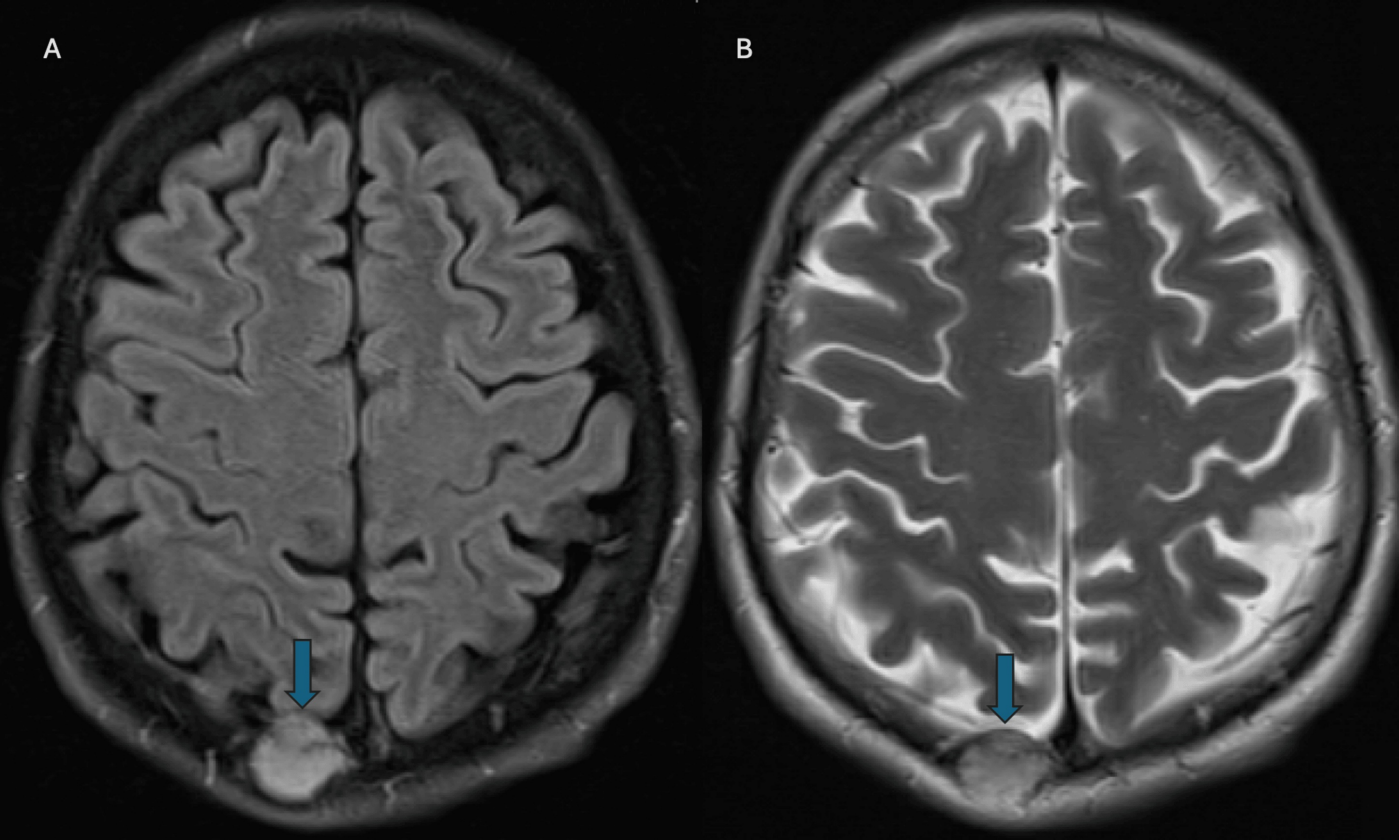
Axial brain MRI demonstrating the primary parietal calvarial meningioma. (A) Axial FLAIR MRI showing a well-circumscribed right parasagittal calvarial mass (arrow) with associated dural involvement and surrounding cortical distortion.
